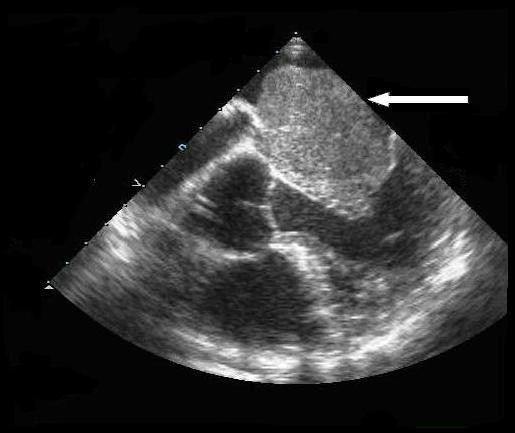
Atrial myxoma: prolapsing through mitral valve. Eleven days pre-operatively, the left atrium and left ventricle are visualized in this transesophageal echocardiogram.
